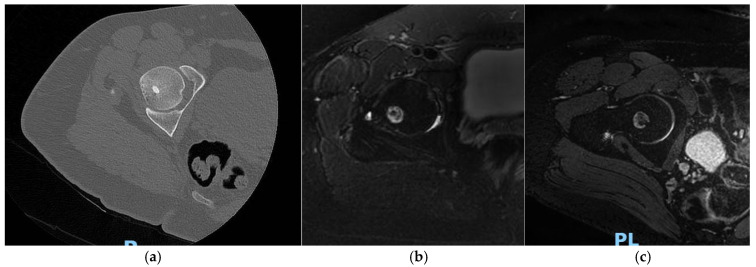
Osteoid osteoma of the femoral head: CT (a) and MRI T2W image with fat saturation (b): perilesional reaction without marrow bone edema; (c) after 6 months, the lesion is dimensionally stable.
